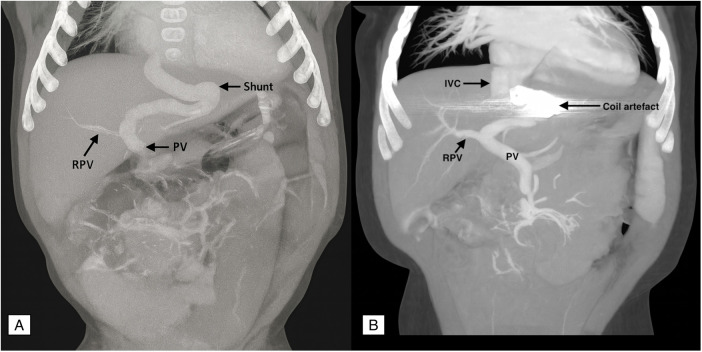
Contrast-enhanced computed tomography. (A) Initial examination revealed a large intrahepatic shunt originating from the left portal vein (PV) branch. The right portal vein branch (RPV) appeared hypoplastic. (B) Follow-up examination 6 months postoperatively demonstrated improved caliber and filling of the right portal vein branch following restoration of hepatopetal portal flow. No residual flow through the shunt was detected. A coil artefact is visible at the embolization site.
